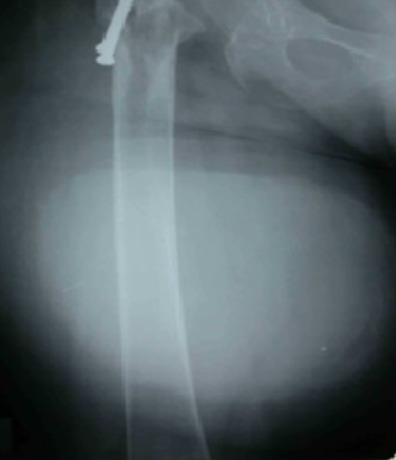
Radiographie de face de la cuisse montrant l'ombre d'une masse
